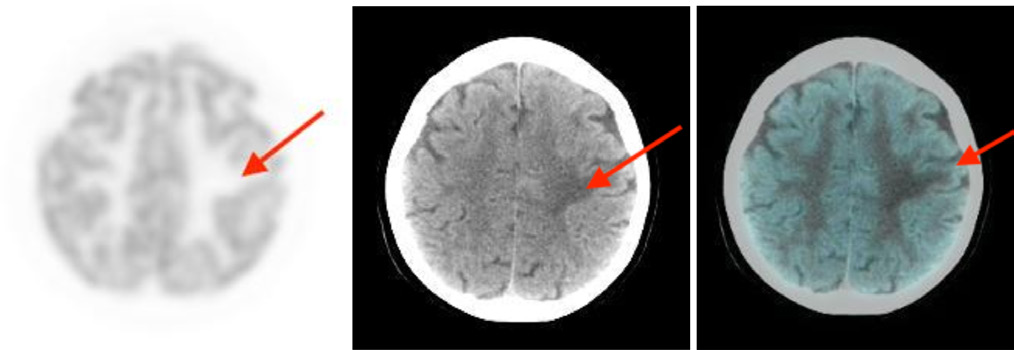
FDG PET (left) showed asymmetrical hypometabolism in left parietal lobe (red arrow) associated with peri-focal oedema on CT (middle).
